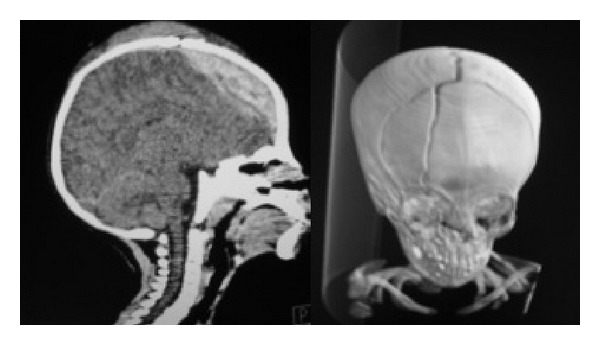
Sagittal and 3D CT scan images showing VEDH and frontal paramedian fracture extending down to the right orbital ceiling.
